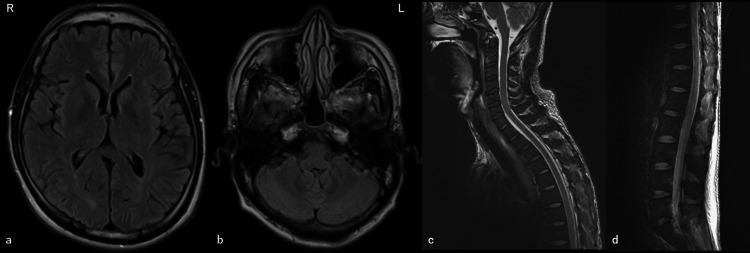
Brain and spinal cord MRI on day 43 after onset. Axial FLAIR of the hemisphere (a, b) and sagittal T2-weighted images of the medulla (c, d). The lesions of the brain and spinal cord had almost disappeared. MRI: magnetic resonance imaging; FLAIR: fluid-attenuated inversion recovery.
